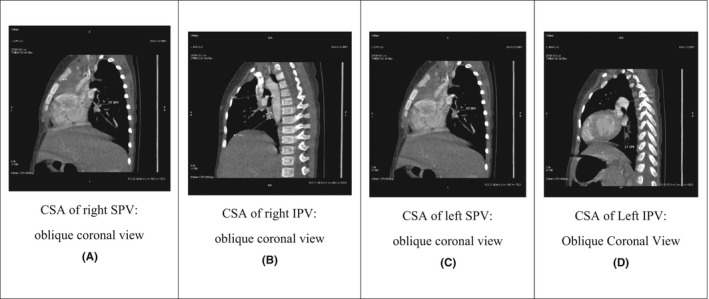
Cardiac CT scan of 1year‐old male child, having Pentalogy of Fallot and right aortic arch (same patient, whose images are shown in Figure 1). Measurements of cross‐sectional area (CSA) of all four pulmonary veins (PV) were taken perpendicular to their lumen about 3 mm before it drains into the left atrium in the oblique coronary images. This patient has a pulmonary vein index (PVI)of 368.12 mm^2^/m^2^
